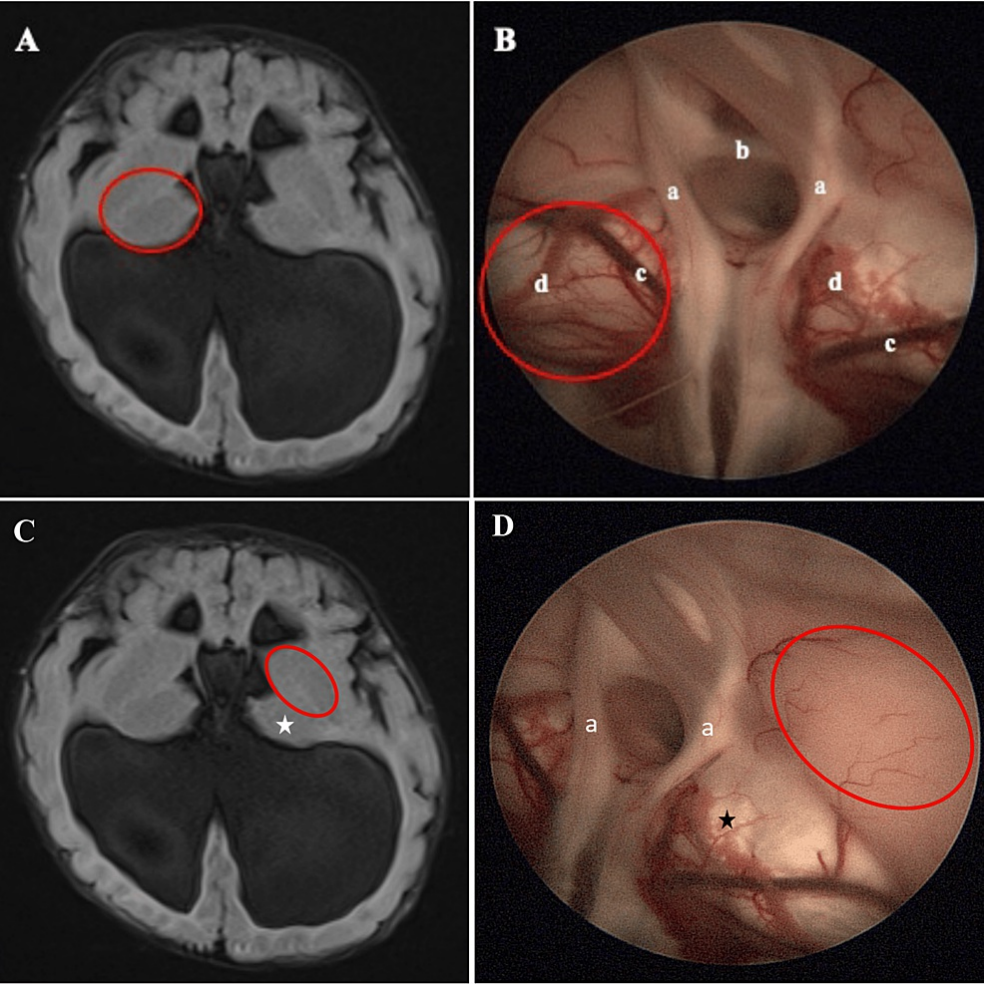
A 6-year-old child with a known Chiari malformation presented with raised intracranial pressure. She was subjected to an endoscopic third ventriculostomy (ETV). The radiology images are of an axial FLAIR MRI brain. (A, B) Due to the absence of septum pellucidum, the endoscope captured the anatomical view on both sides of the hemisphere. The red circle is an area of the thalamus that appears yellowish. (C, D) However, further antero-superior-lateral, the striatum appears slightly brownish (red circle). Star, thalamus; a, bilateral fornices; b, anterior commissure; c, thalamostriate vein; d, choroid plexus.
